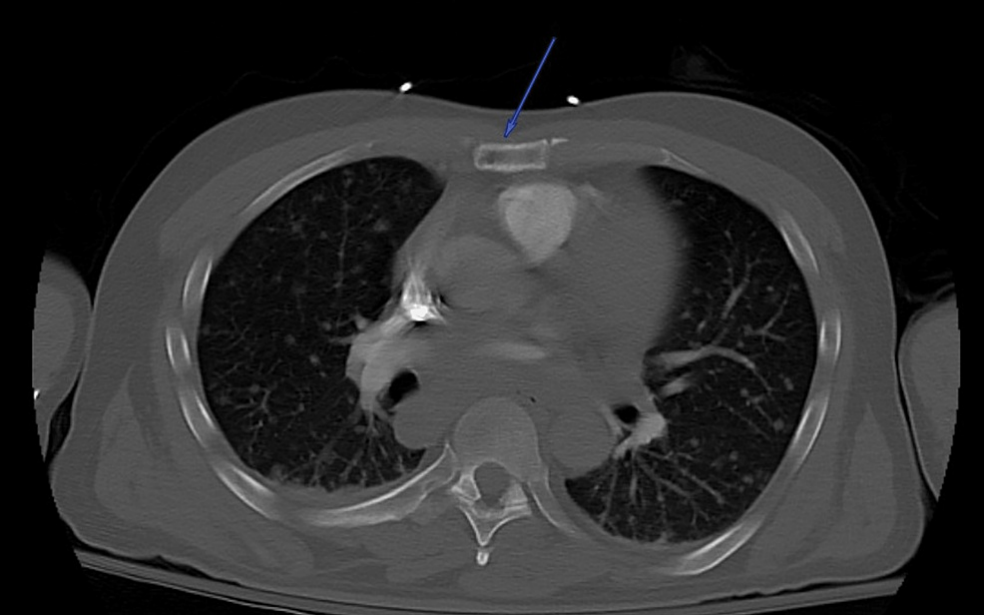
Axial bone window CTA scan of the chest revealing a lytic sternal lesion (blue arrow) CTA: computed tomography angiography
